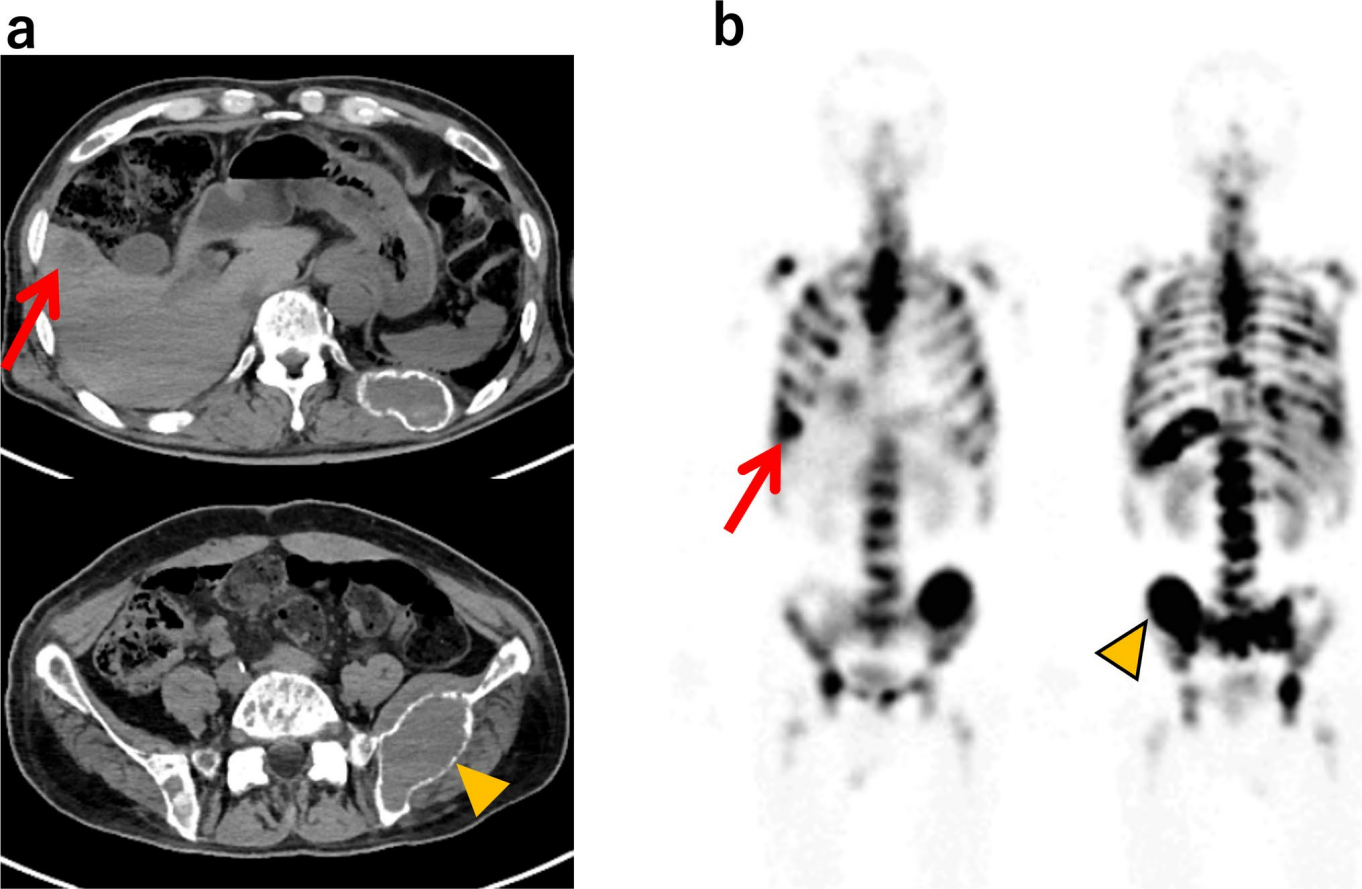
A case of incomplete ^177^Lu-DOTATATE treatment. a CT axial view, b
